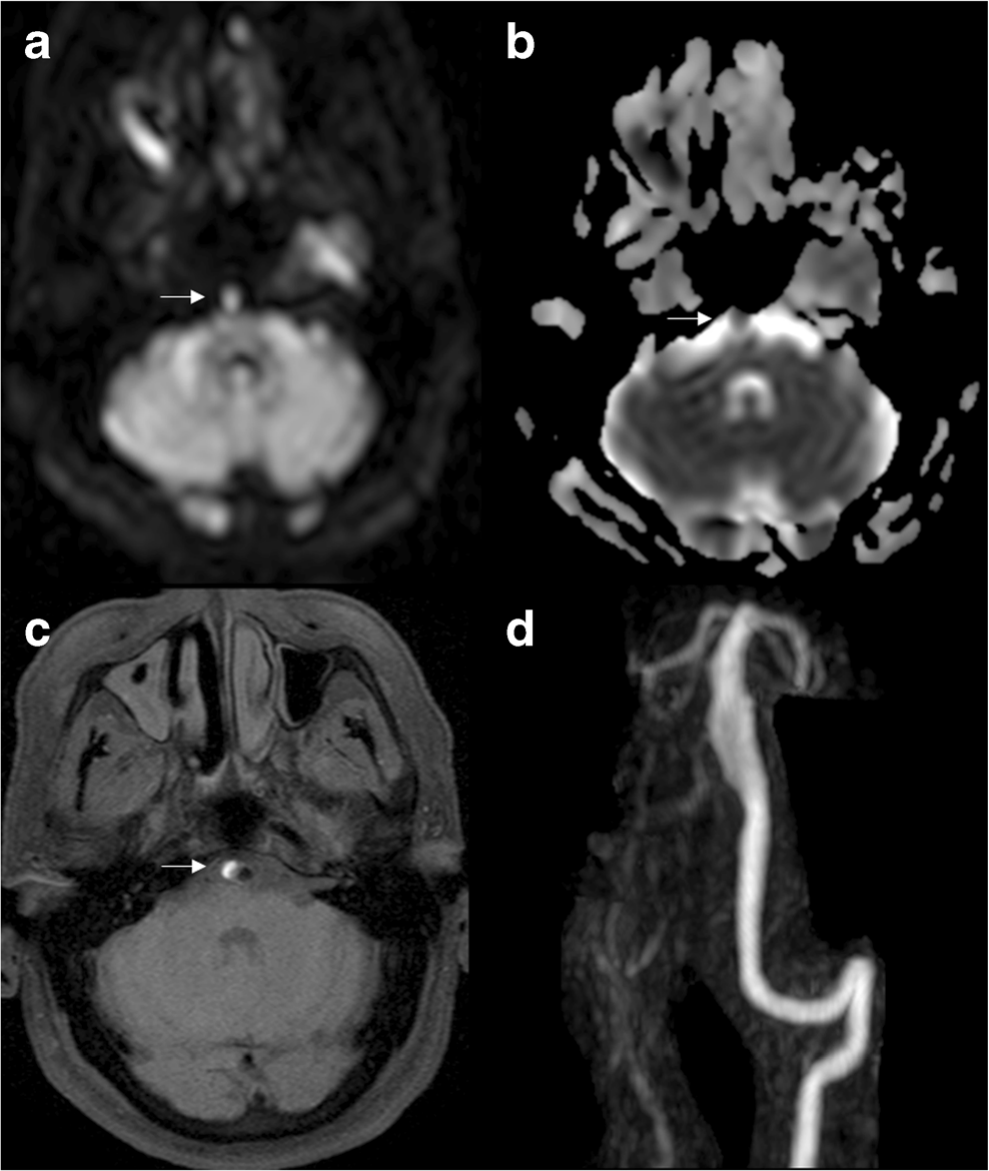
Arterial dissection. DWI (a), ADC (b), non-contrast FAT SAT T1 (c) and coronal MIP reconstruction of the basilar artery (d) demonstrate a dissection with mural haematoma represented by the “crescent sign” (arrows), which shows restricted diffusion and hyperintensity on T1WI
